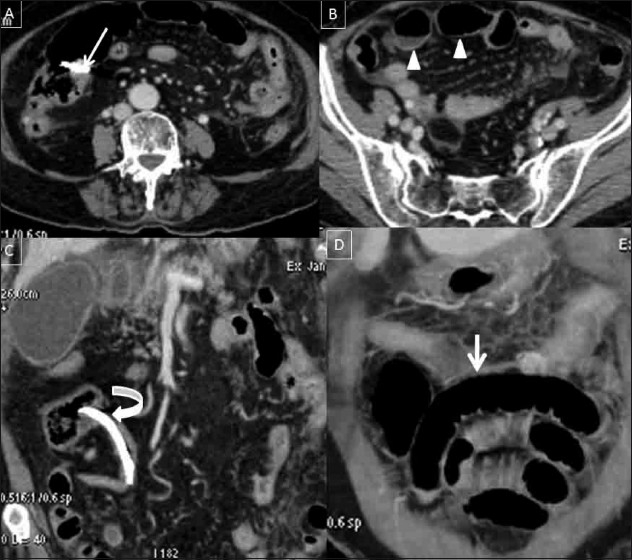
Axial (A,B) and coronal reformatted (C,D) contrastenhanced CT scans of the abdomen and pelvis show the migrated stent entrapped at the ileocecal junction (white arrow in A), protruding into the cecal lumen (curved arrow in c), with resultant dilatation of the small bowel loops with mild fluid accumulation (white arrow heads in B, arrows in D). The distal small bowel loops are edematous with inflammatory fat stranding in the adjacent mesentery
